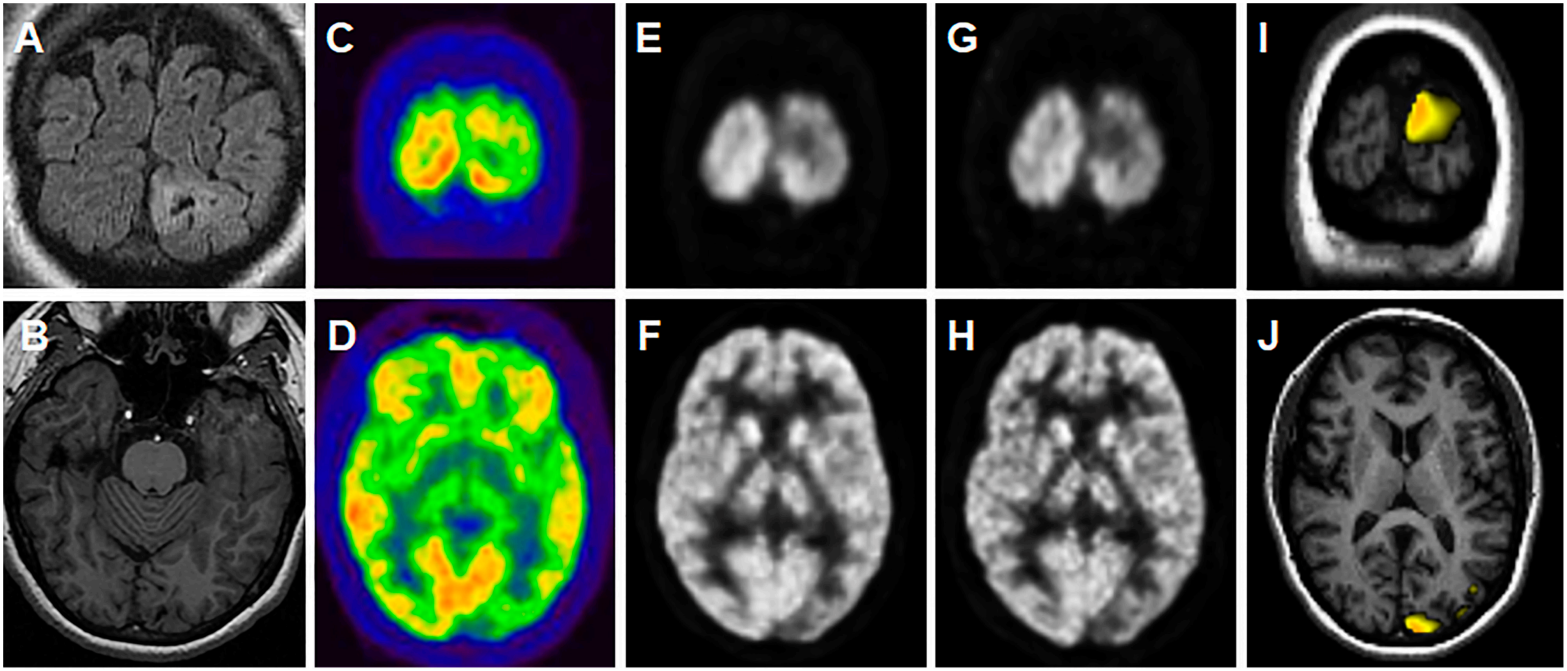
A representative case of the utility of PET-analysis. Patient with a 34-year history of medically refractory epilepsy with weekly seizures (Table 2, patient 16). Video-EEG showed epileptiform activity in the left occipital region. MRI depicted a focal lesion in the left occipital lobe (A,B) which was suggestive of a small area of residual encephalomalacia or secondly, cortical dysplasia with subcortical extension (MRI sequences from up to down: coronal FLAIR and axial contrast-enhanced T1-weighted). On suspicion of dysplasia, a PET study was requested to plan the extent of surgical resection, and a left occipital hypometabolism was seen on visual assessment (C,D). No hypometabolic areas were present at any threshold in SPM analysis, although a left occipital hypometabolism was seen in the SPM-normalized images (E,F), which was more evident in the PET-analysis-normalized images (G,H). A left occipital hypometabolic area appeared on PET-analysis (thresholds shown: *p* = 0.008 and k = 200), corresponding with the occipital lesion on corregistered images with T1-weighted MRI (I,J). The lesion was surgically resected and the pathology study diagnosed a chronic hemorrhagic necrosis foci with gliosis. Fifty-nine months years after surgery the patient is completely seizure-free (Engel I).
